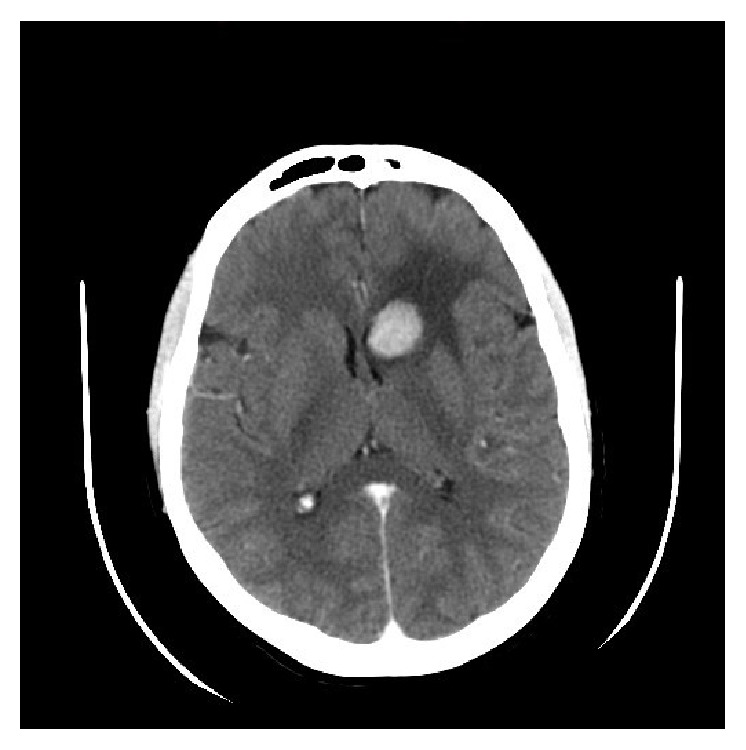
Enhanced axial CT scan of the brain shows multiple enhancing brain lesions seen supratentorially and infratentorially; the largest is seen in left caudate head with surrounding reactive parenchymal edema.
